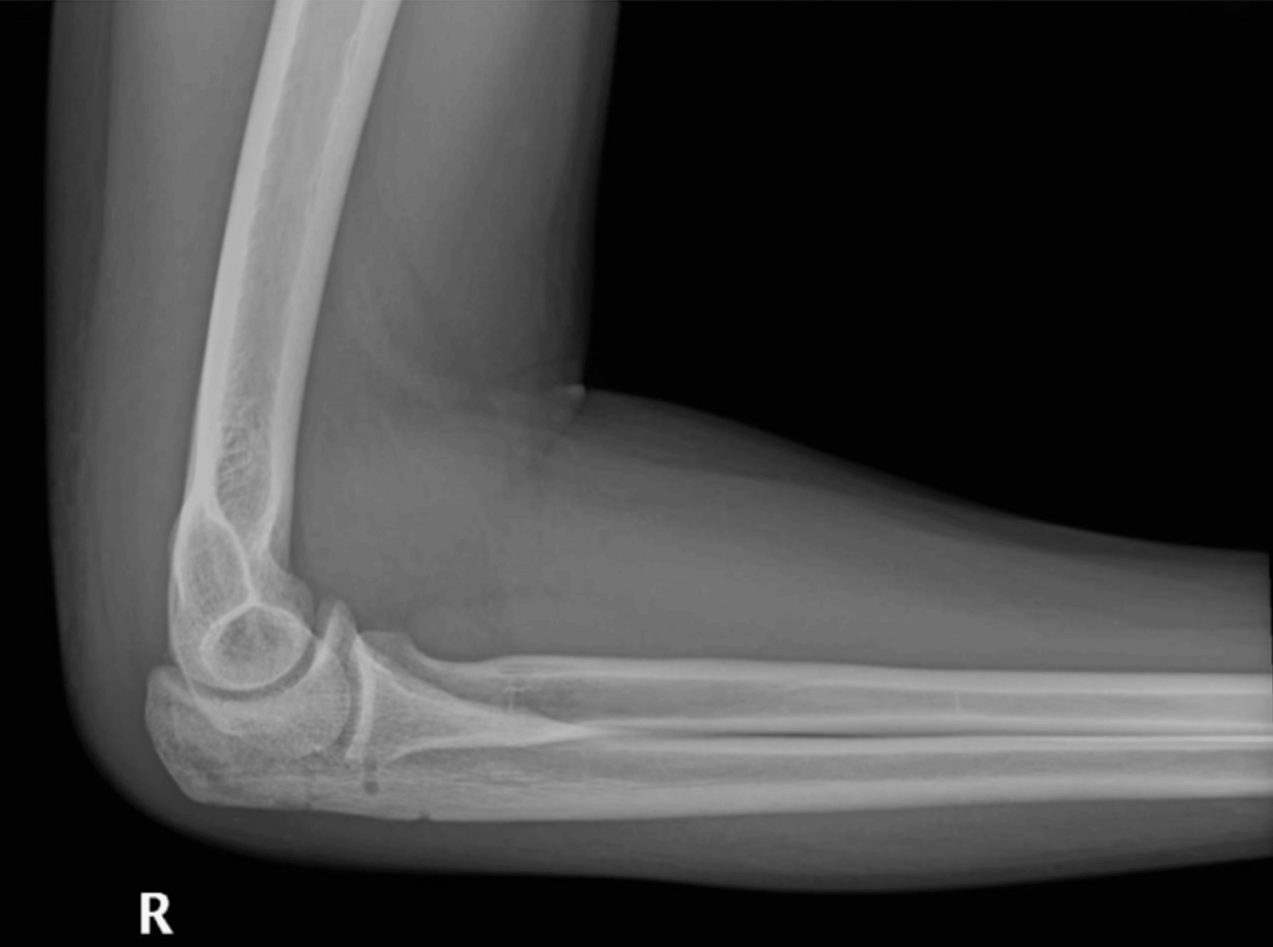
Six weeks postoperative lateral X-ray image of the right elbow.
